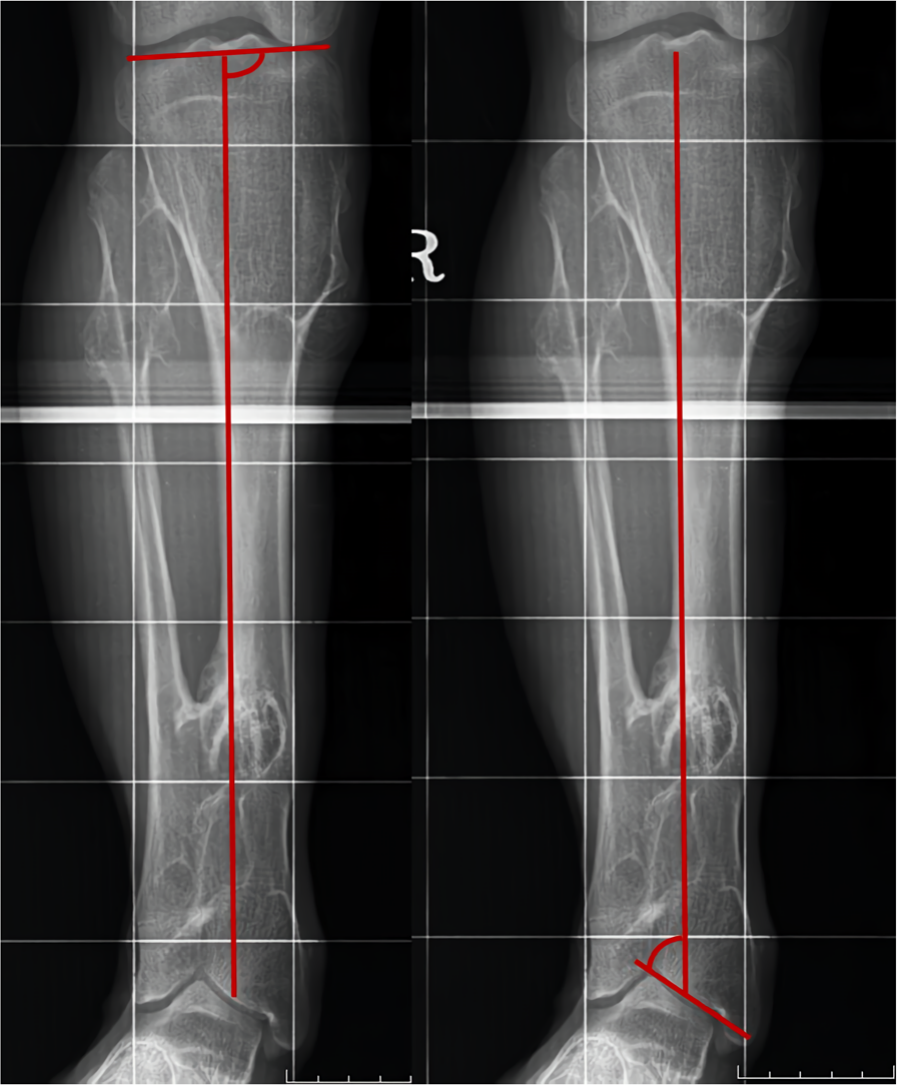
Measurement of radiographic angle of MPTA and LDTA. The MPTA is determined by measuring the angle created by a line of the central axis of the tibia and a second line drawn across the proximal tibial epiphyseal surface or joint line. In a similar way, LDTA is measured by the angle created by a line of central axis of the tibia and a second line drawn across the distal tibial epiphyseal surface or talar plafond
